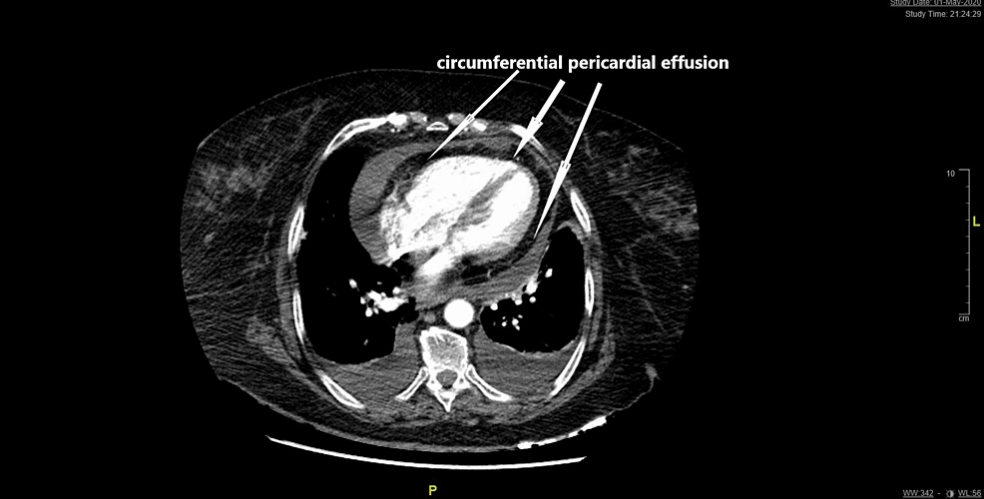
CT chest showing moderate circumferential pericardial effusion
